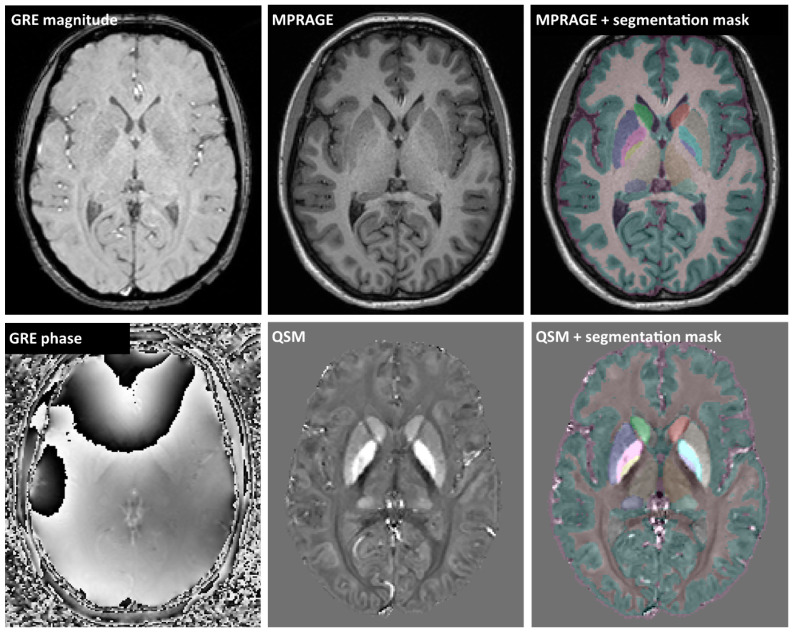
Example MRI images: gradient-echo (GRE) magnitude and phase images were processed to quantitative susceptibility map (QSM). Multi-atlas segmentation employed coregistered QSM and MPRAGE images to generate masks of deep grey matter structures, cerebral white and grey matter, and CSF.
